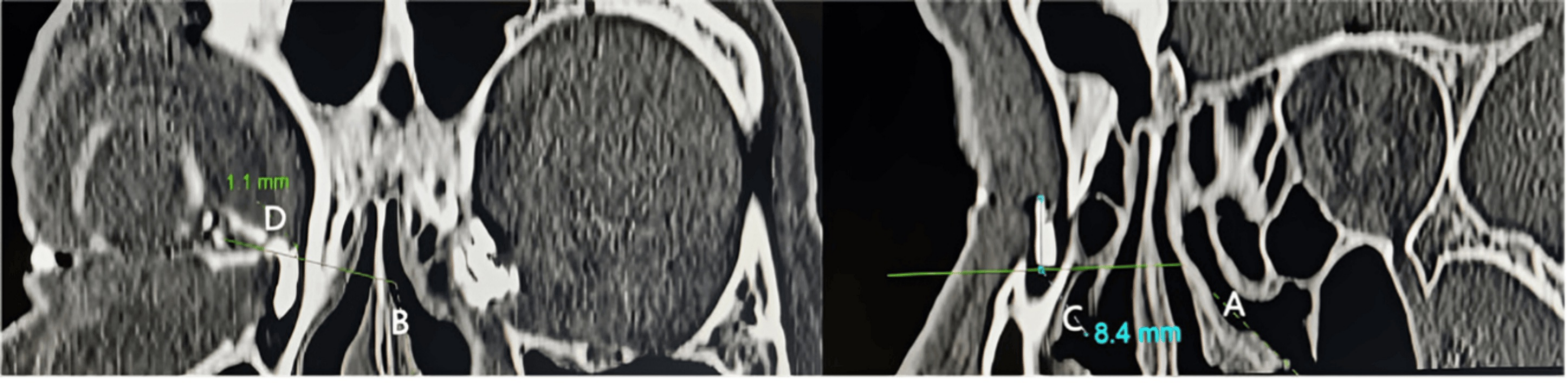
Parasagittal computed tomographic dacryocystography (CT-DCG) images showing measurements along the long axis of a small lacrimal sac. In this approach, the distance between the middle turbinate axilla (MTA) and the common canaliculus (CC) is indirectly calculated, as both structures are rarely visible simultaneously on coronal images. A vertical line (A) is drawn perpendicularly from the MTA to the long axis of the sac, while another perpendicular line (B) is drawn from the CC to the same axis. The height of the sac above the MTA (C) and above the CC (D) are then determined. The distance between the MTA and CC is obtained by subtracting the height D from height C. Figure from Yang et al., licensed under CC BY-NC-ND 4.0 [18].
